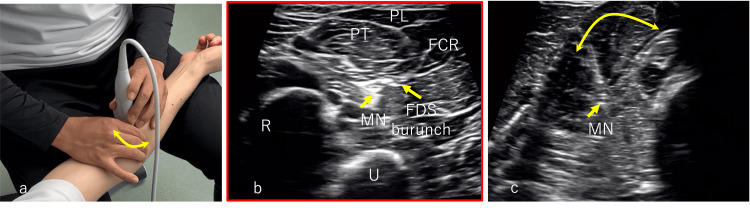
Ultrasound-guided exercise of the right median nerve (a) Approach image,(b) Ultrasound image at rest,(c) Ultrasound image during manual intervention to enhance neural gliding.Yellow double arrow: Direction of manual manipulation (displacement of the FDS and surrounding area of the median nerve in the short-axis direction relative to the forearm).Yellow single arrow: deformation of the median nerve FDS branch and surrounding muscles induced by manual manipulation.R, radius; U, ulna; PT, pronator teres; PL, palmaris longus; FCR, flexor carpi radialis; FDS, flexor digitorum superficialis; FCU, flexor carpi ulnaris; FDP, flexor digitorum profundus; MN, median nerve; FDS branch, branch of the flexor digitorum superficialis
